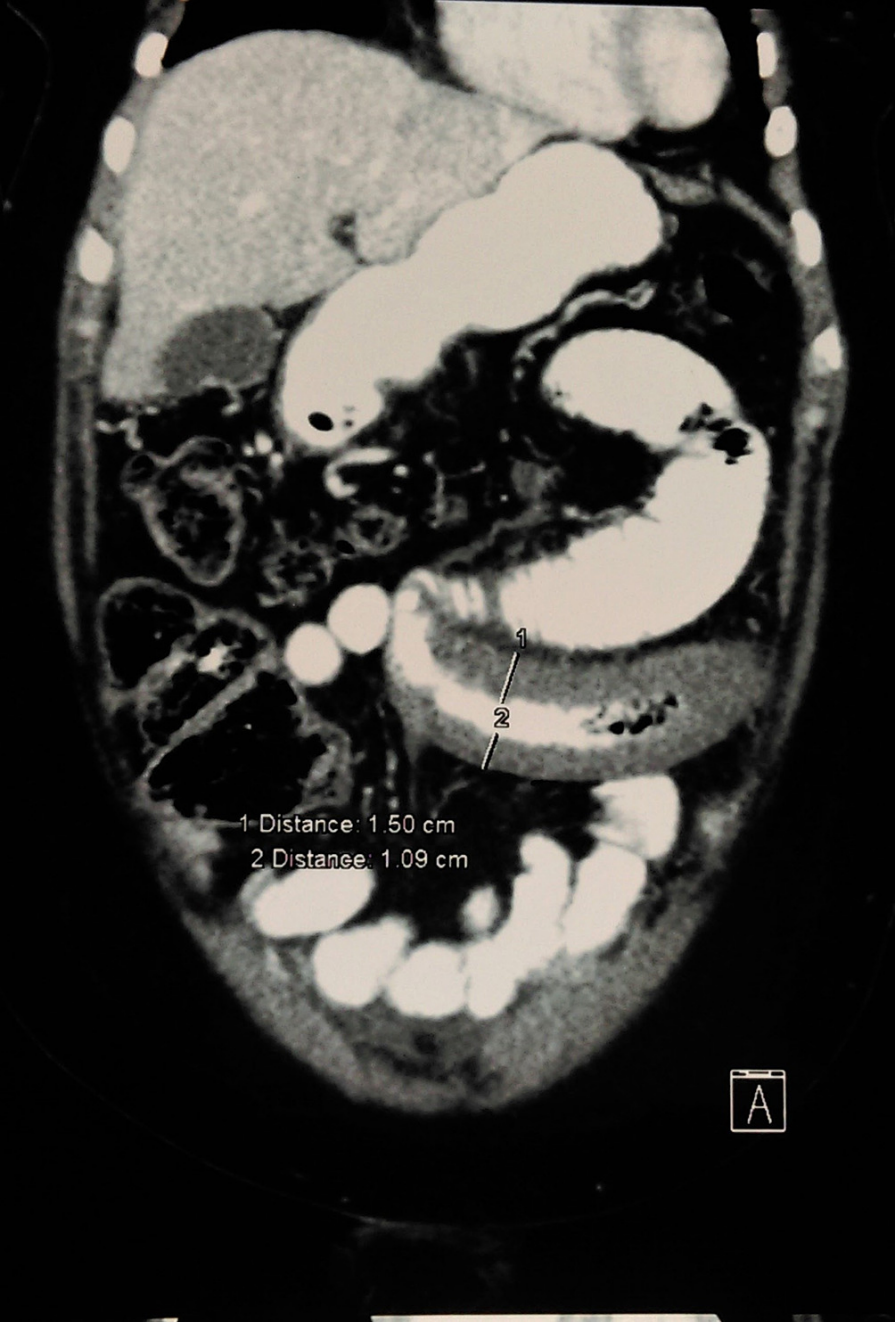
CT coronal reformatted image shows acute segmental jejunal infarction with smooth concentric non enhancing wall thickening.
